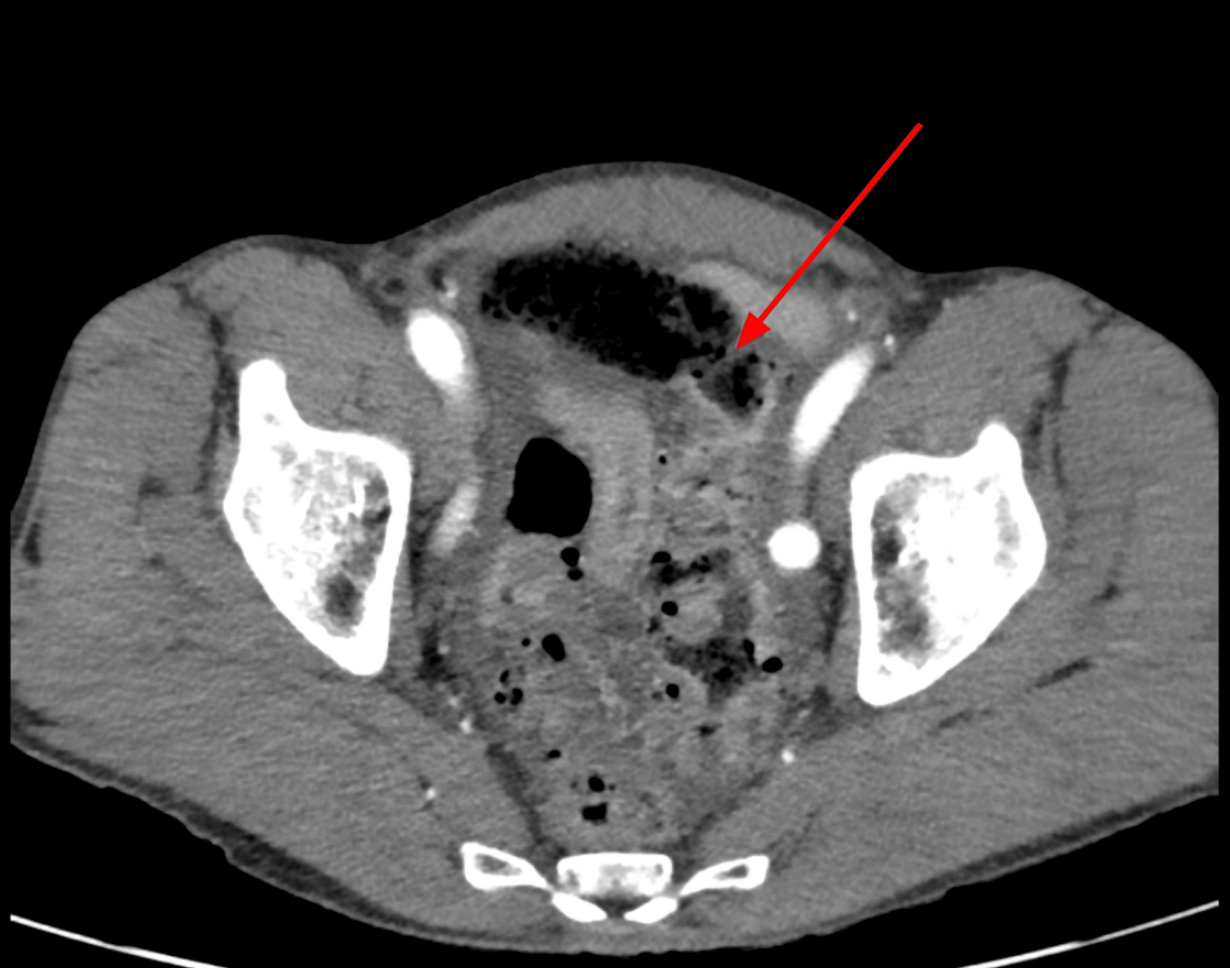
CT in the transverse plane showing a large perforation of the proximal sigmoid colon (red arrow) with feculent material extending into the anterior pelvis
